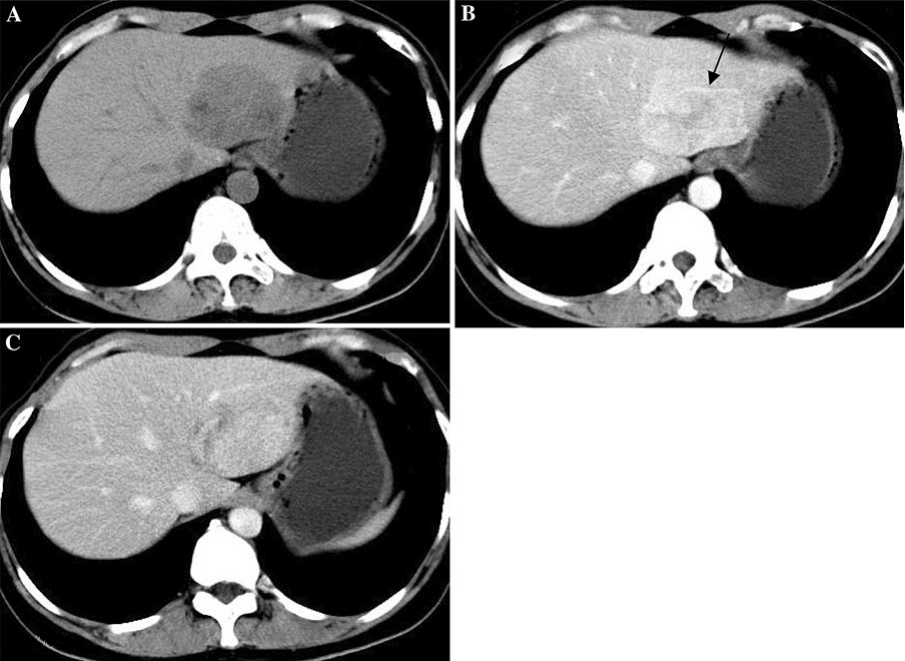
43-Year-old woman with epithelioid AML in left lobe of liver (patient 2). A Non-enhanced CT scan shows hypoattenuating lesion in segment 2/3. B Contrast-enhanced CT scan shows obviously enhanced lesion in the arterial phase; filiform vessels (*arrow*) within the lesion is show in the arterial phase image. C The lesion is isoattenuating in the portal venous phase.
